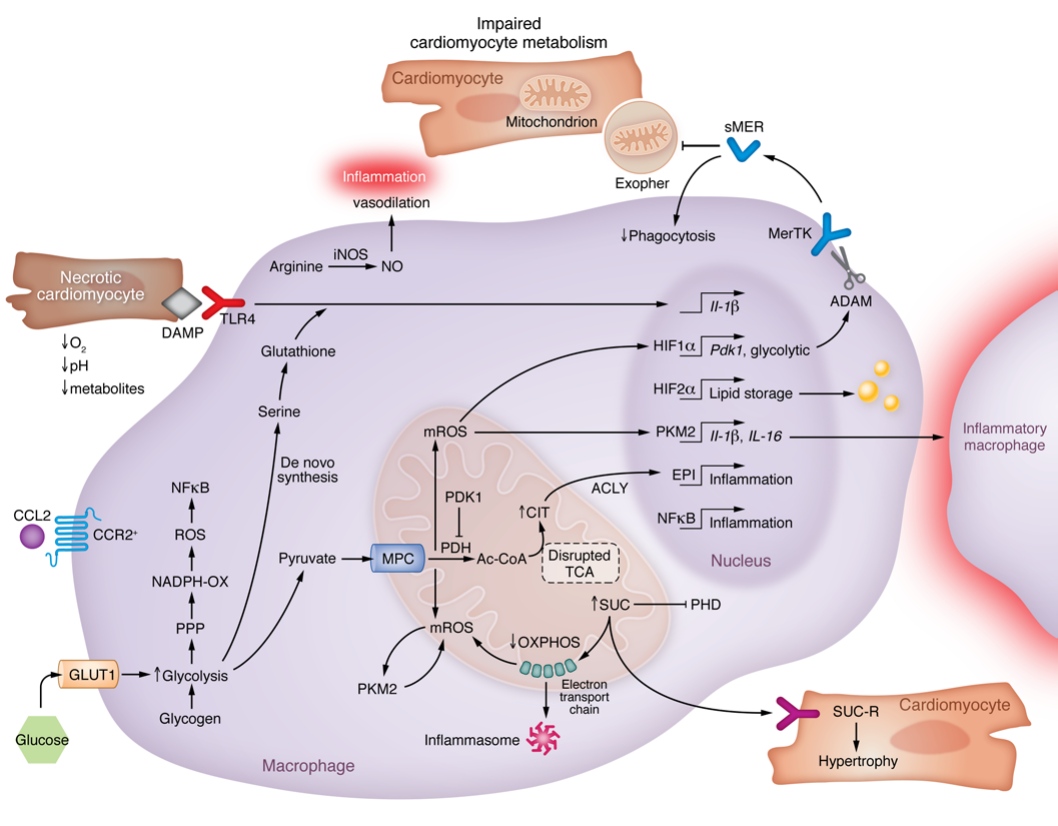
Working model of inflammatory cardiac macrophage metabolism after MI. Inflammatory immunometabolic reactions of cardiac macrophages are implicated in the response after MI. In CCR2^+^ macrophages, glucose utilization (detected by ^18^FDG-PET) through GLUT1 fuels glycolysis and inflammatory pathways including ROS, mROS, and other intermediates that promote inflammation. Metabolic HIF-1α activation may induce a disintegrin and metalloproteinase (ADAM) proteases and lead to proteolysis of phagocytic receptors such as MerTK to suppress phagocytosis of exophers and dying cells. Altered mitochondrial metabolism is also associated with the accumulation of TCA intermediates (such as succinate [SUC]) that may signal intercellular crosstalk and have the capacity to alter epigenetic regulation of proinflammatory cytokine genes. ACLY, ATP citrate lyase; CCL2, also known as monocyte chemoattractant protein-1 (MCP-1); CIT, citrate; DAMP, damage-associated molecular pattern; EPI, epigenetic; FDG, fluorodeoxyglucose; GLUT1, glucose transporter protein type 1, also known as SLC2A1; HIF-1α, hypoxia-inducible factor 1α; MPC, mitochondrial pyruvate carrier; mROS, mitochondrial reactive oxygen species; OXPHOS, oxidative phosphorylation; PDH, pyruvate dehydrogenase; PKM2, pyruvate kinase M2; PPP, pentose phosphate pathway; SDH, succinate dehydrogenase; TCA, tricarboxylic acid; TLR, Toll-like receptor.
